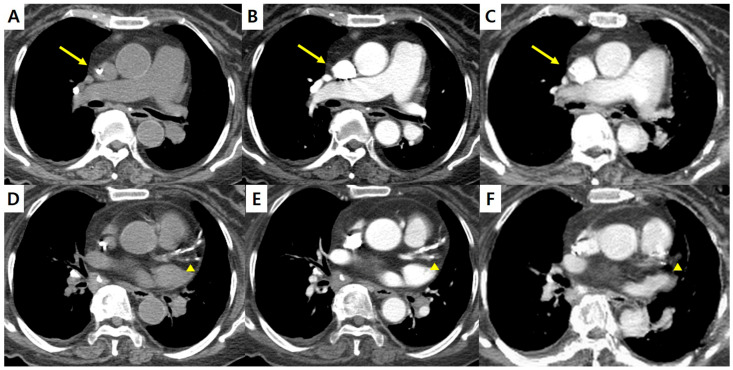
Chest CT images of a 76-year-old woman with lymphoma. (A,D) NCCT, (B,E) CECT, and (C,F) sCECT. sCECT demonstrates reduced beam-hardening artifacts caused by medical devices in the superior vena cava (arrows). Coronary artery calcifications (arrowheads) appear less conspicuous on sCECT compared with NCCT and CECT. CECT = contrast-enhanced CT; NCCT = non-contrast CT; sCECT = synthetic contrast-enhanced CT.
